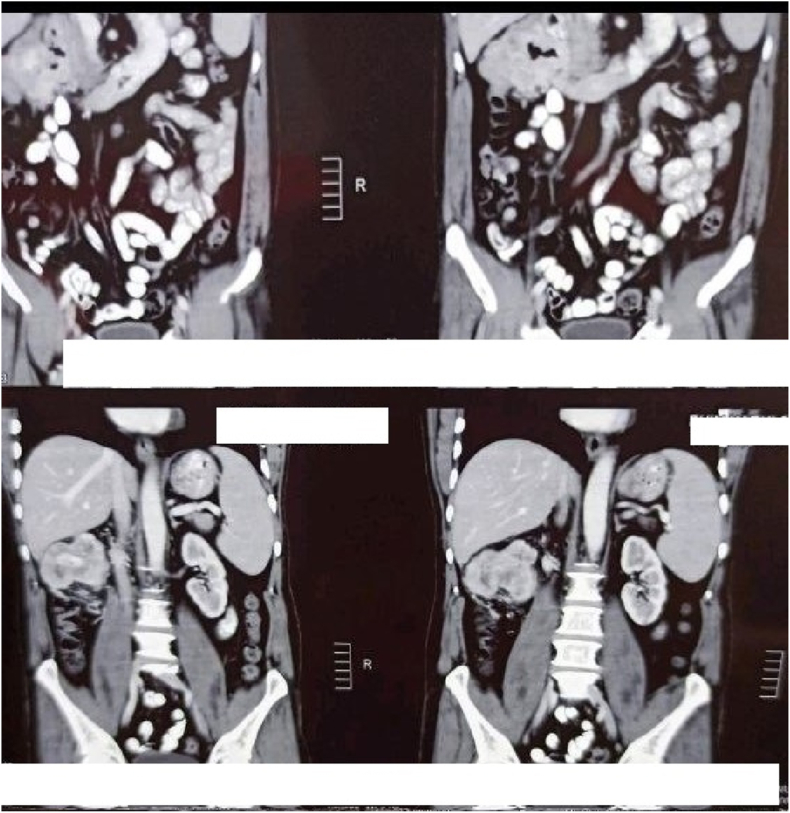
CT abdomen showing encapsulated mass extending to inferior surface of liver (coronal section).
